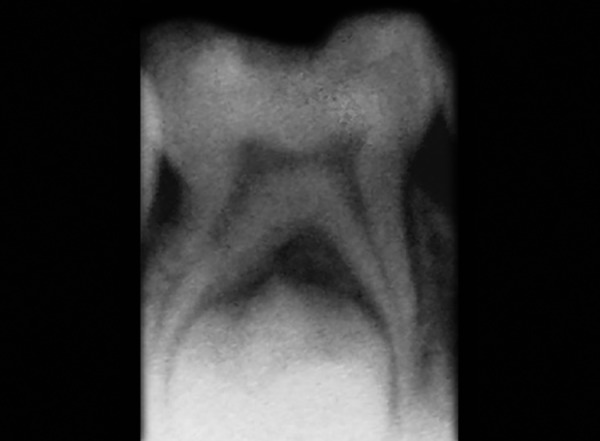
Preoperative radiograph of 85
